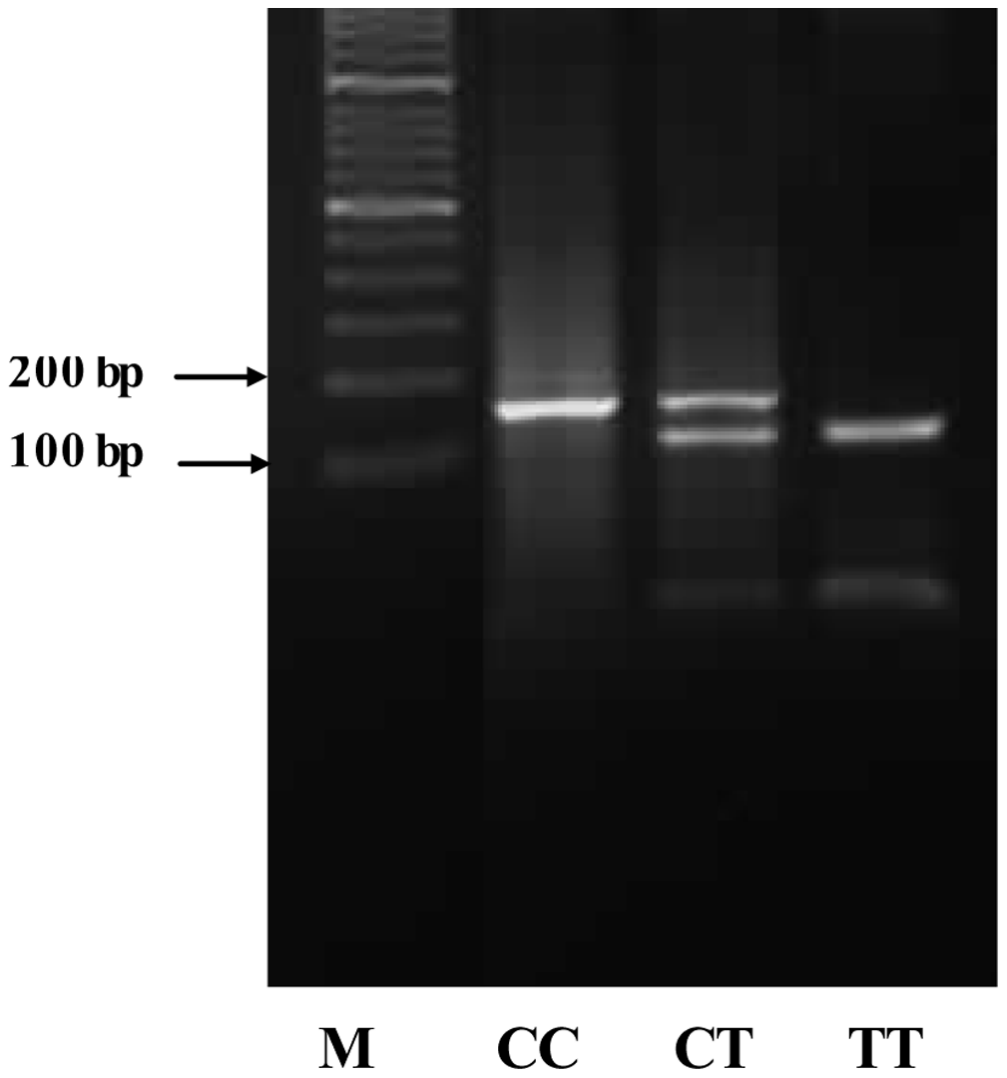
The image of genotyping result for rs290487. Line 1: Maker; Line 2: CC genotype-153 bp; Line 3: CT genotype-153/128 bp; Line4: TT genotype-128 bp.
